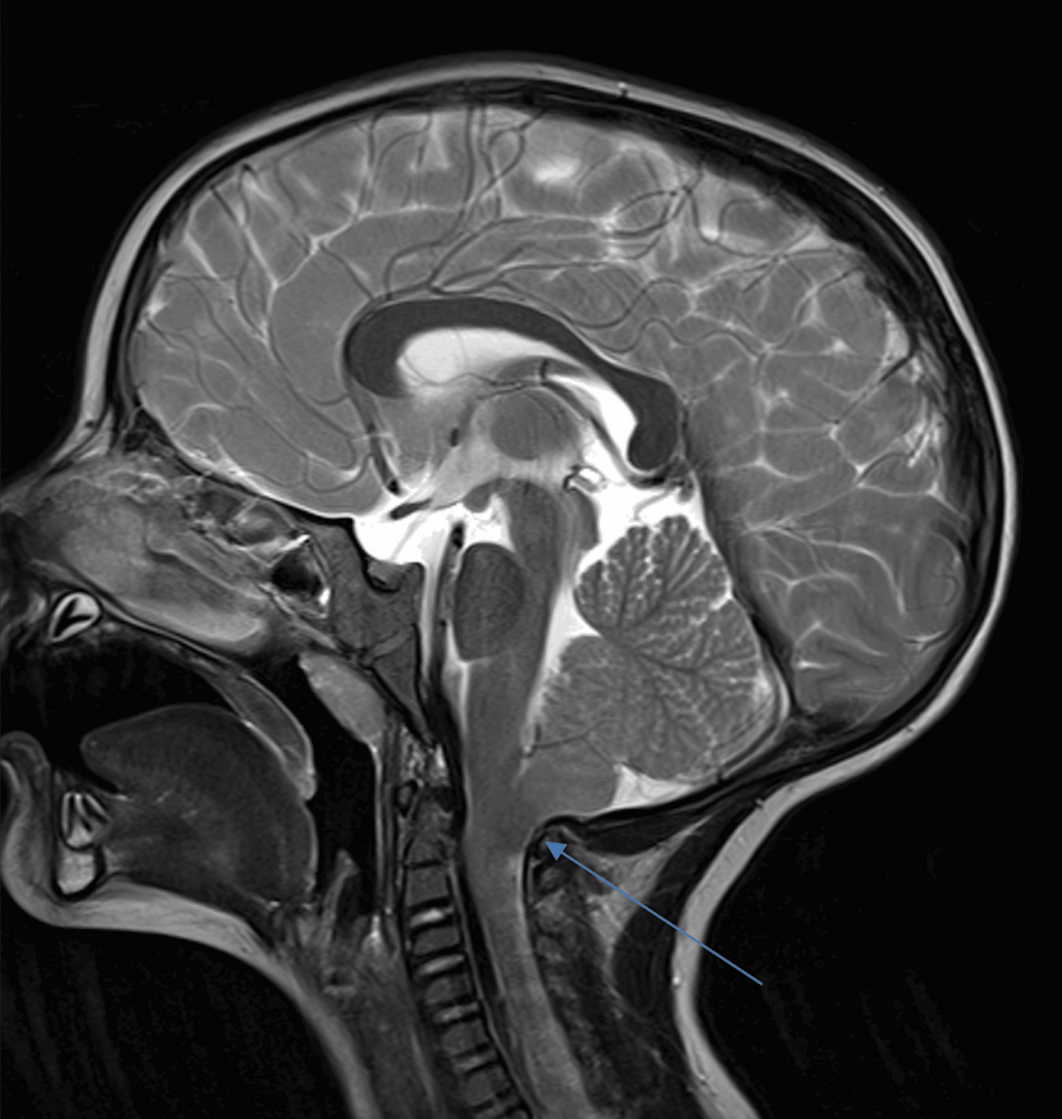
Preoperative sagittal T2 MRI imaging of the proband. Blue arrow indicates herniation of cerebellar tonsils at the foramen magnum.
